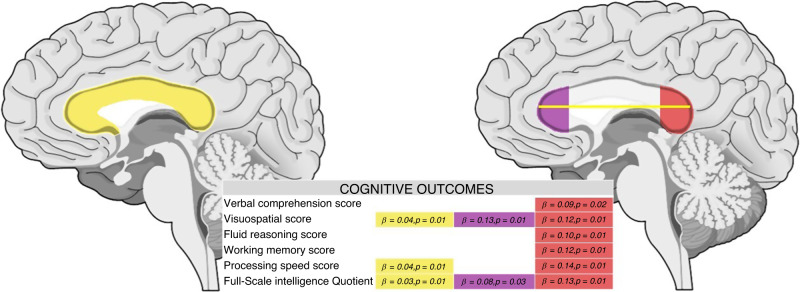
CC areas related with motor and cognitive subscales. All models were adjusted by GA at birth, sex and age at MRI. Only statistically significant results were expressed in the tables. CC corpus callosum, GA gestational age, MRI magnetic resonance imaging.
